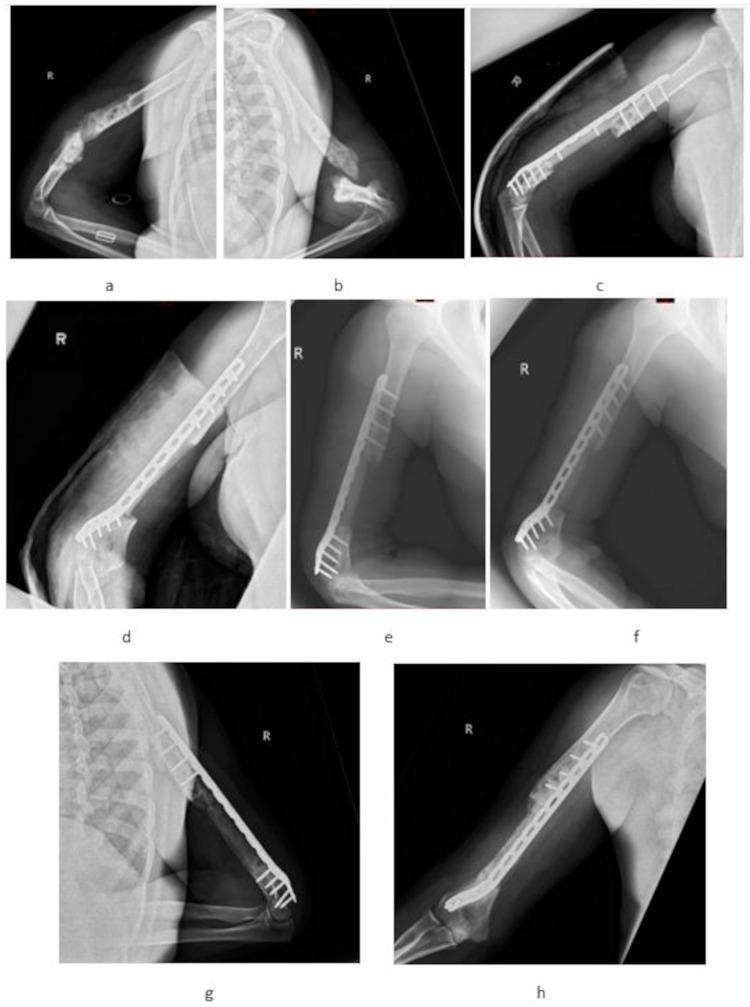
A 48-year-old female with post-traumatic pseudoarthritis of the right humerus (5 years after injury). (a,b) preoperative X-rays; (c,d) first-stage IMT postoperative X-ray humerus stabilization with long titanium LCP with PMMA (with clindamycin, gentamycin, vancomycin); (e,f) second-stage IMT postoperative X-ray autologous femoral RIA graft input to vascularized induced membrane; (g,h) healthy humerus bone and radiological union.
